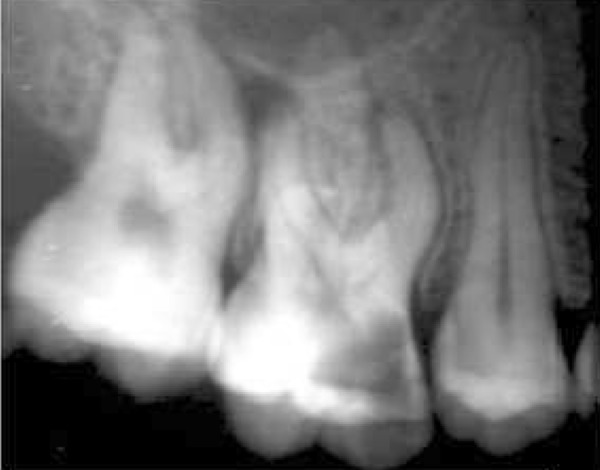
The initial radiograph of tooth #16
